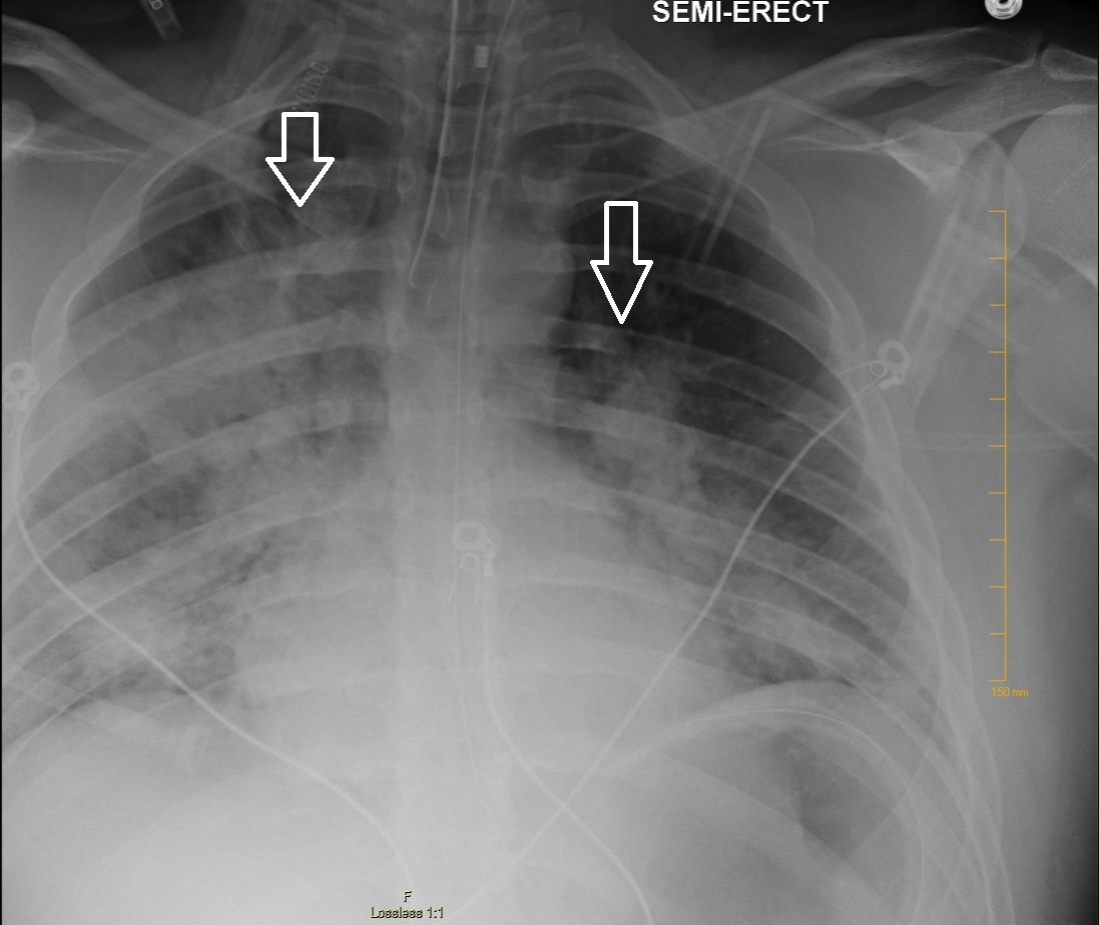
Chest radiography demonstrating bilateral hilar opacities (arrows)
